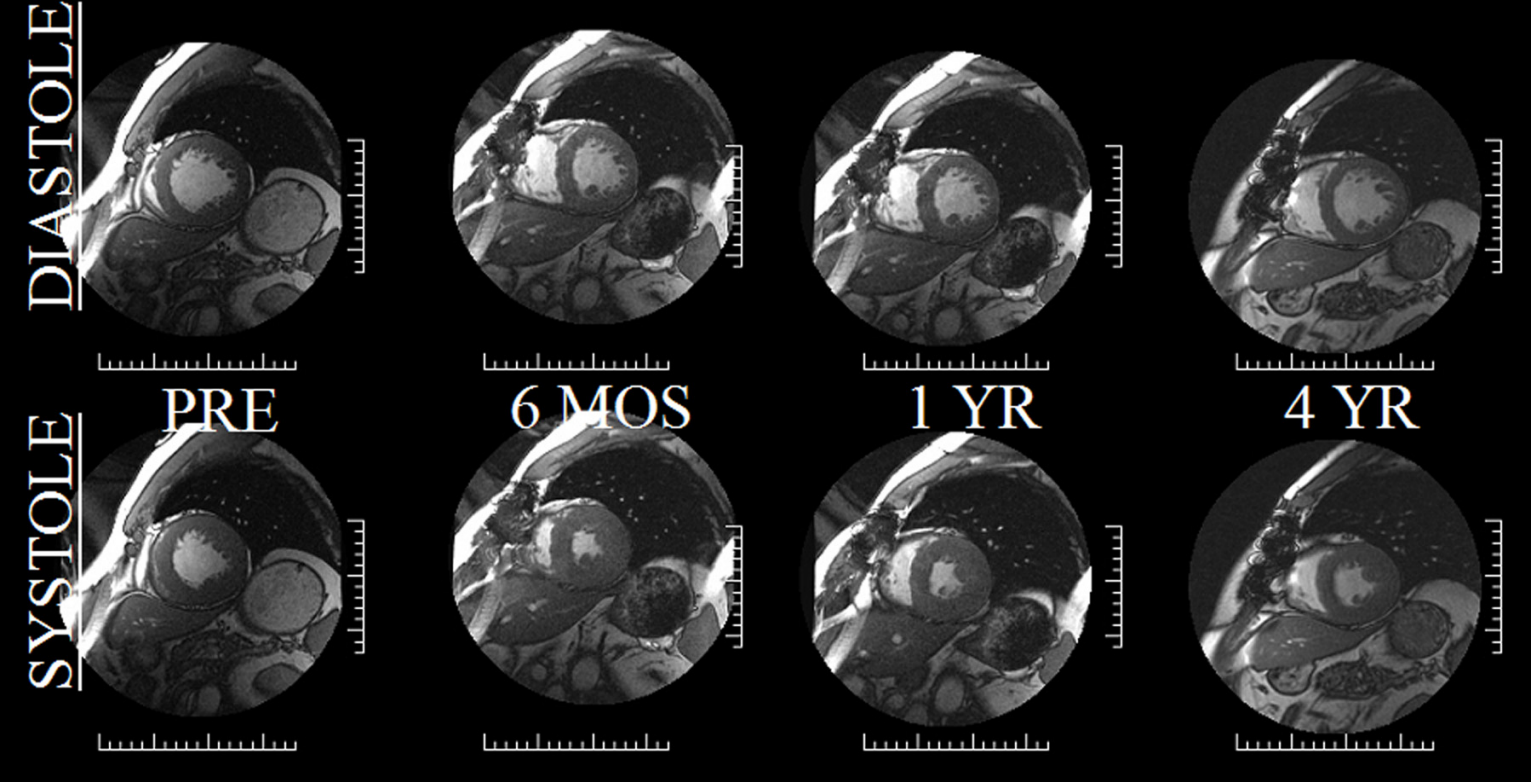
Serial cardiovascular MRI mid short-axis images in diastole (top row) and systole (bottom row) in a 76 WM taken the day prior to AVR, 6 months, one year and 4 years following AVR. The LV mass decreased from 186 to 154 g over the first 6 months to only regress to 132 g over the next 3 1/2 years demonstrating the early-rapid and late-slow pattern of LVH regression. Similarly, LVEF markedly improved after afterload relief from 54% to 60% in the first 6 months with no further improvements over the ensuing 3 1/2 years (62%).
